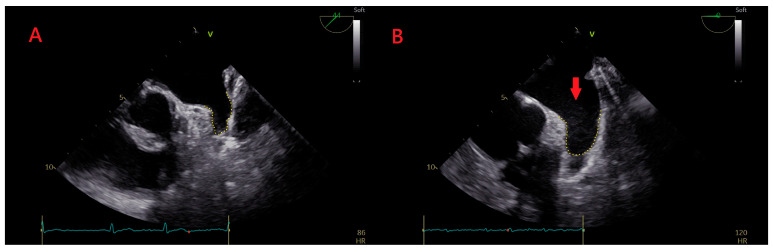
Representative echocardiogram of LASEC. (A) No LASEC was detected by TEE. (B) LASEC (arrow) was revealed by TEE. V stands for left side, numbers 0 and 44 stand for imaging plane angle.
